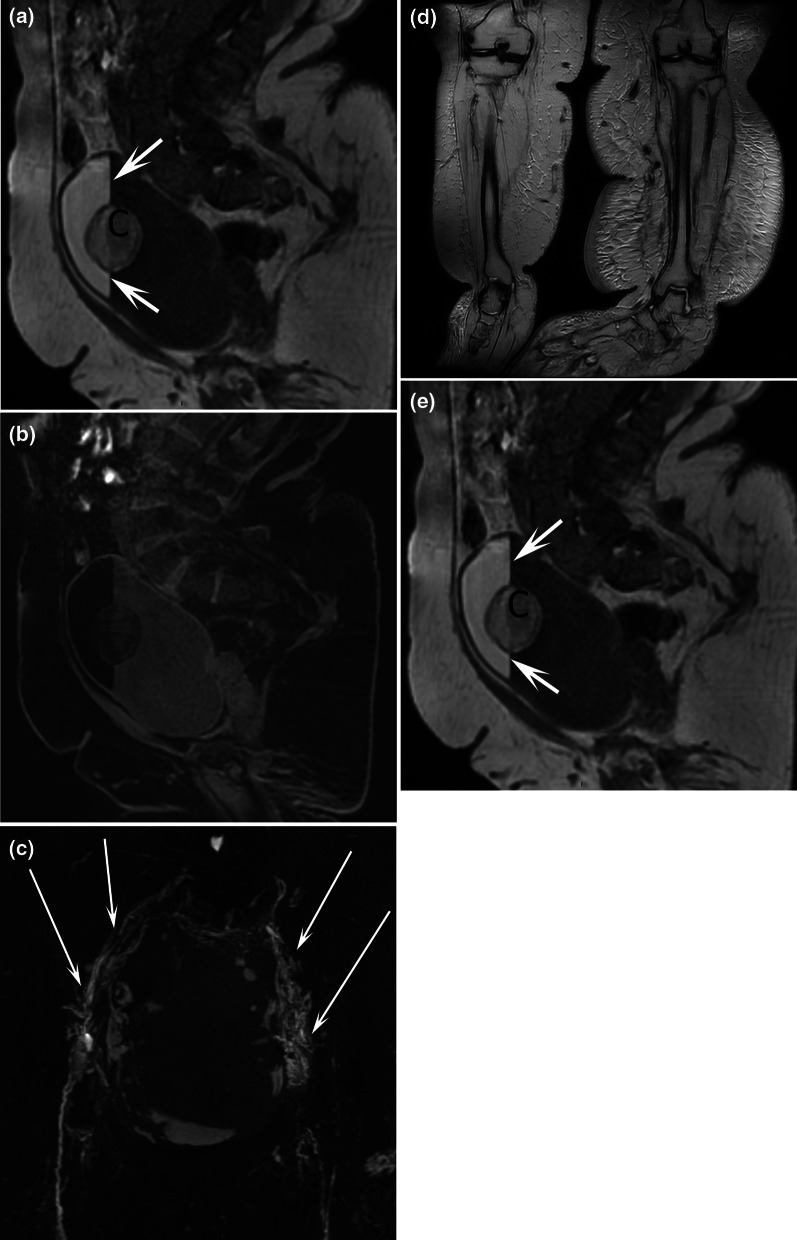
44-year-old female patient with chyluria. Sagittal T2-weighted MR image (a) and T1-weighted MR image with fat saturation (b) demonstrated an intravesical fat-fluid level (arrows) and chylous clot (C). Coronal MR lymphography with MIP reconstruction c demonstrated a channel type lymphatic malformation (arrows) in touch with both faces of the bladder. Coronal MR lymphography with MIP reconstruction (d) demonstrated bilateral lower limb lymphedema (L) that was more severe on the left side with dilatation of lymphatic vessels (arrows). Corresponding coronal T2-weighted MR image (e) showed bilateral congenital deformities of the ankles and feet
